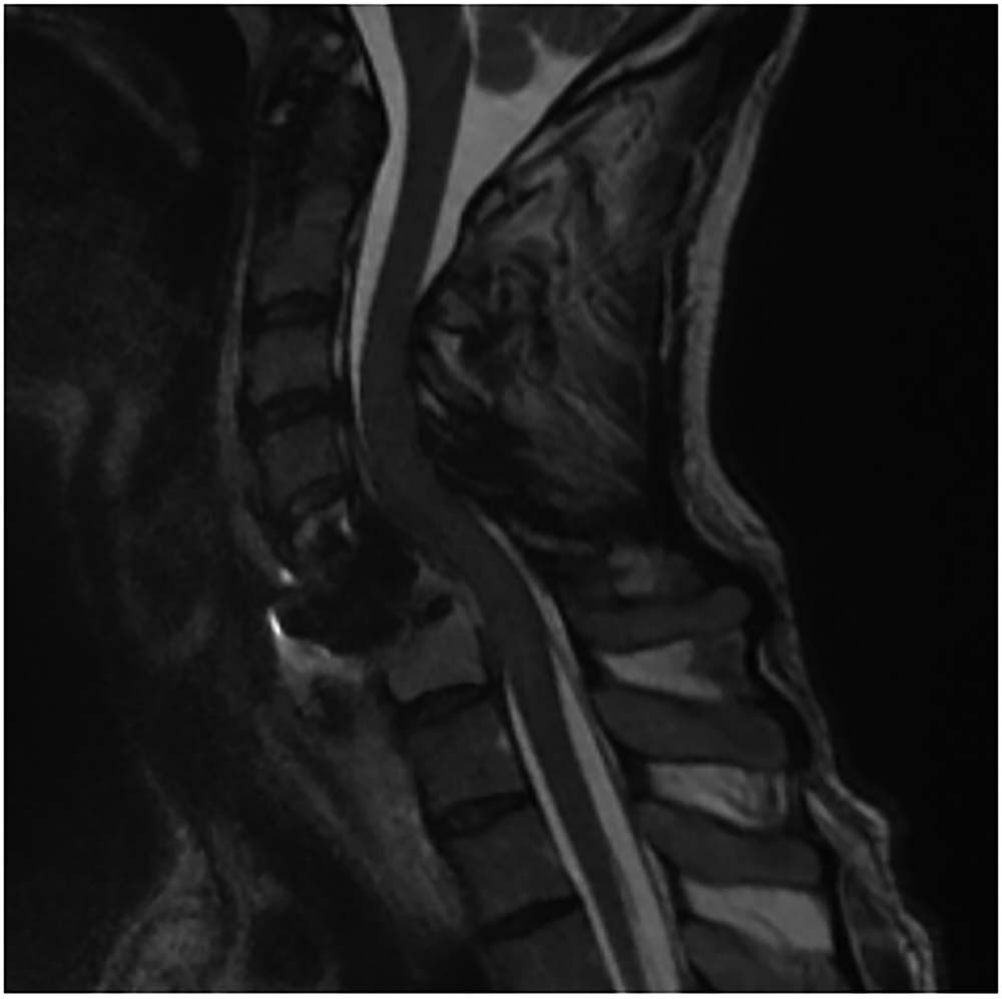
Sagittal T2-weighted MR of prior retropharyngeal abscess that led to revision and posterior plating from C5 to T1.
